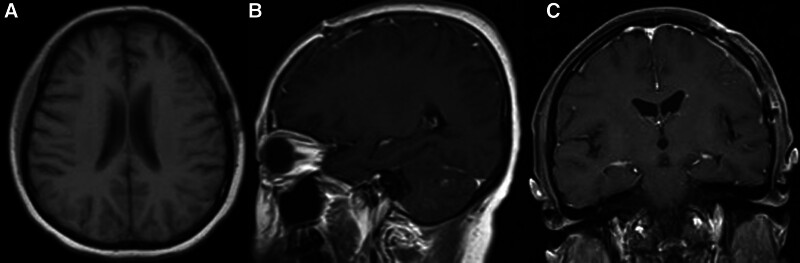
(A–C) Eleven months after surgery, a follow-up MRI revealed no signs of tumor recurrence.
